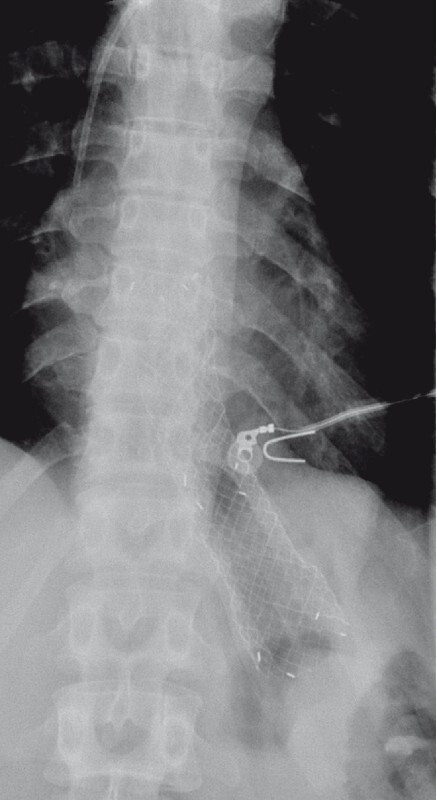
Radiograph showing the migrated self-expandable metal stent (SEMS). It was not possible to pass the endoscope through it.
